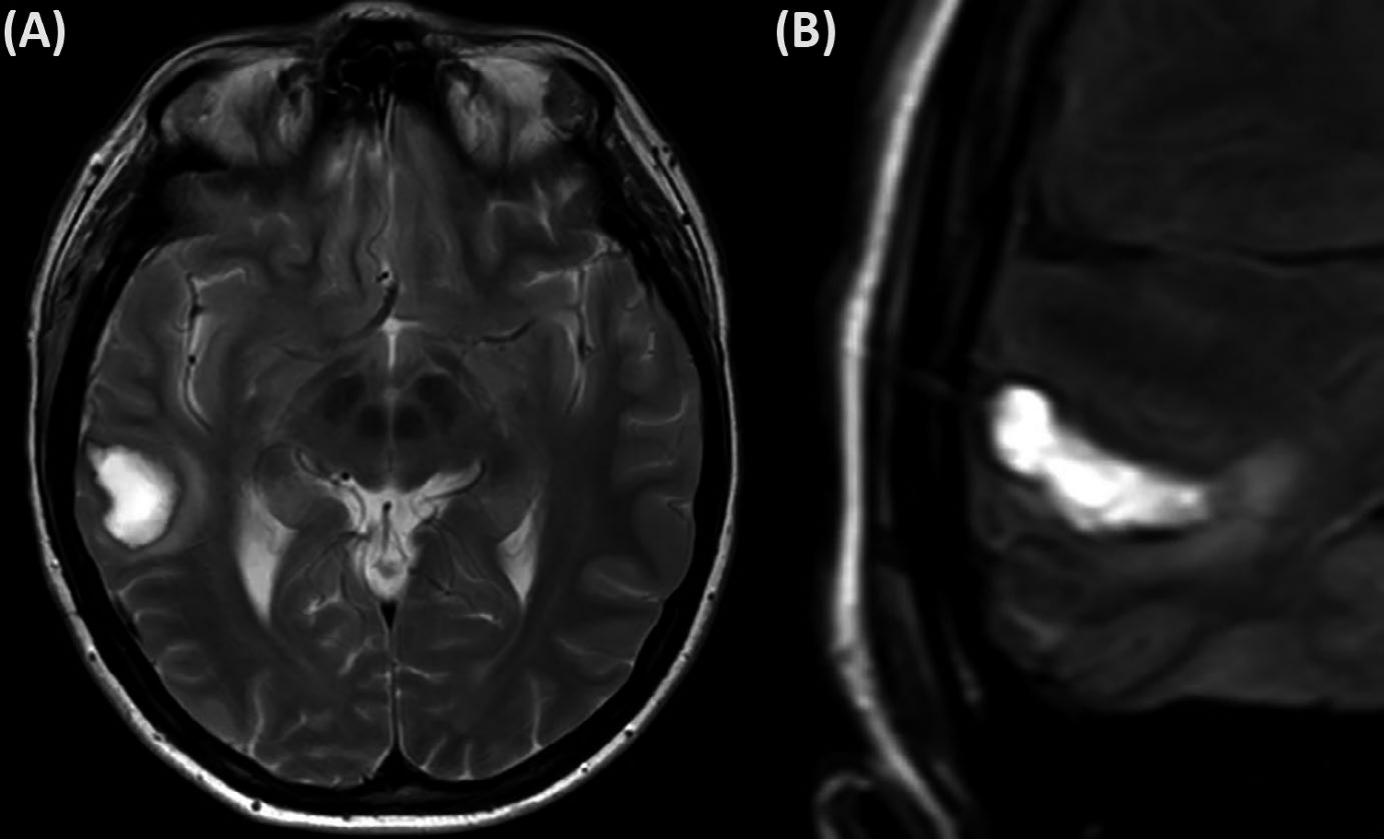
Neuroimaging changes consistent with intracranial hemorrhage. T2 MRI (A) and FLAIR (B) of the brain demonstrate a hematoma in the right temporal lobe
